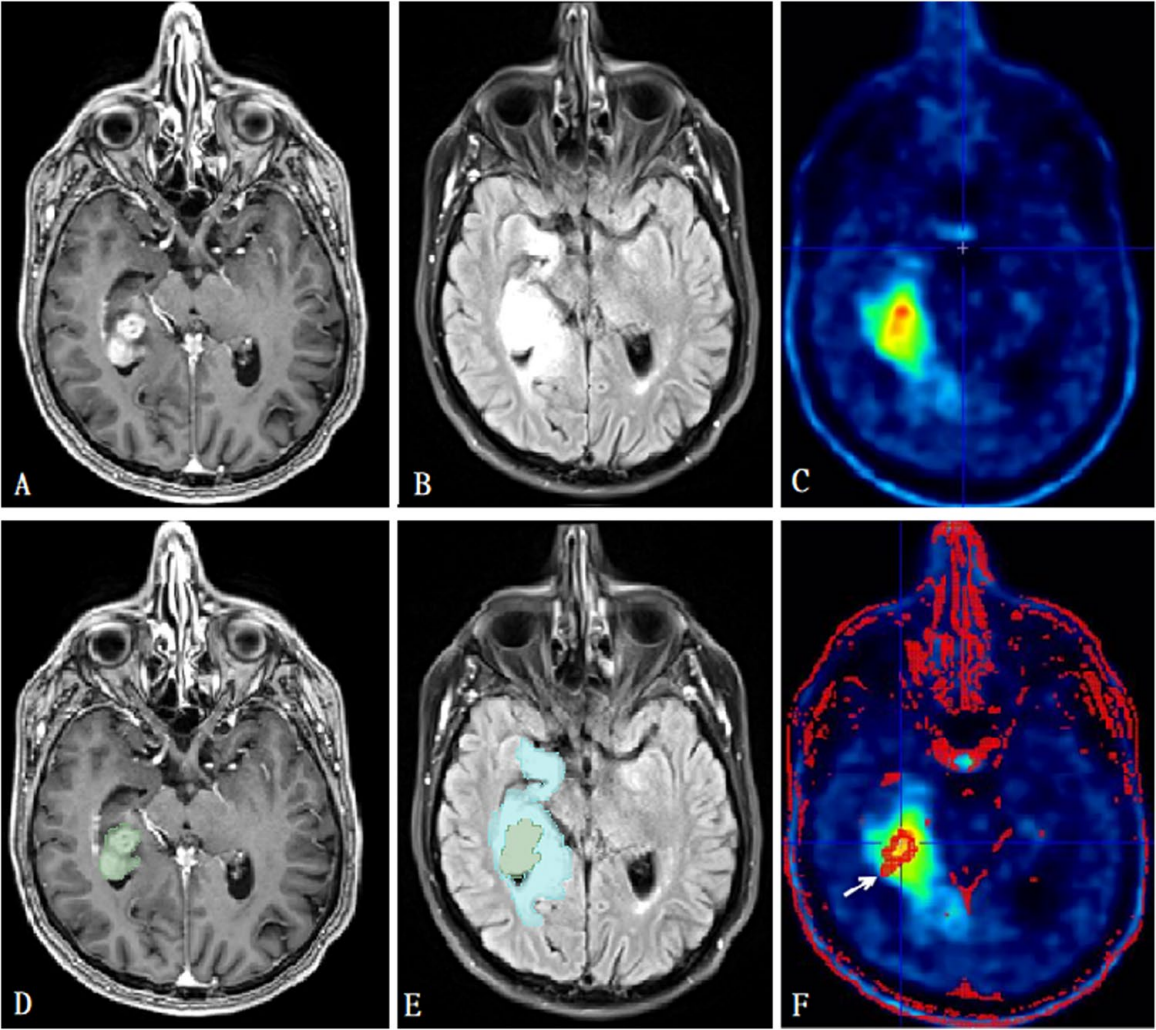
Segmentation of volumes of interest. A Post-contrast T1-weighted MRI before segmentation demonstrating a right mesiotemporal ventricle-contacting GBM. B FLAIR sequence of the same patient before segmentation. C metPET in the same patient before masking with the MRI images demonstrating increased uptake. D The same slice as in A, but after segmentation of the contrast enhancing lesion (green mask). E The same slice as in B, but after peritumoral edema segmentation (blue mask). F The same slice as in C, but after segmentation. The mask based on the contrast enhancing lesion is indicated with an arrow. If necessary, masking was adjusted to match manual segmentations
